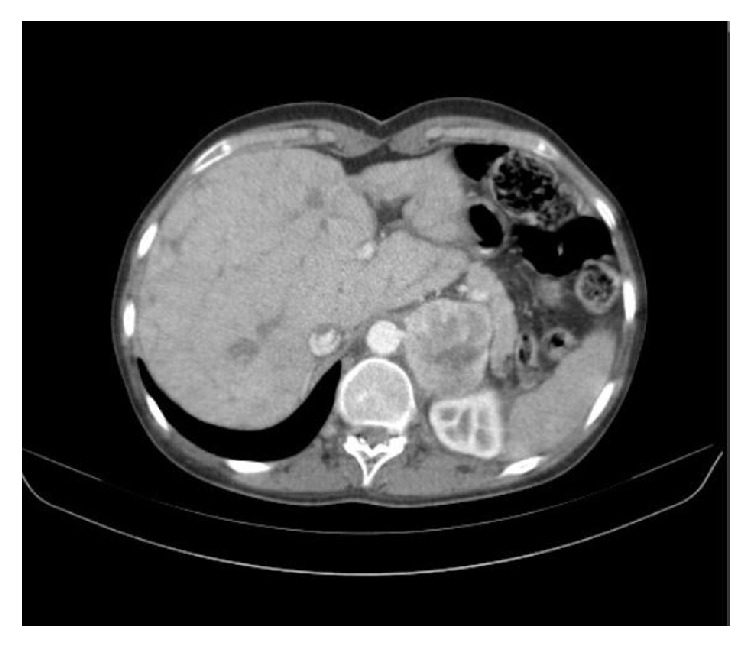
Abdominal CT scan: presence of a big mass in the left adrenal lodge (6-cm mass).
